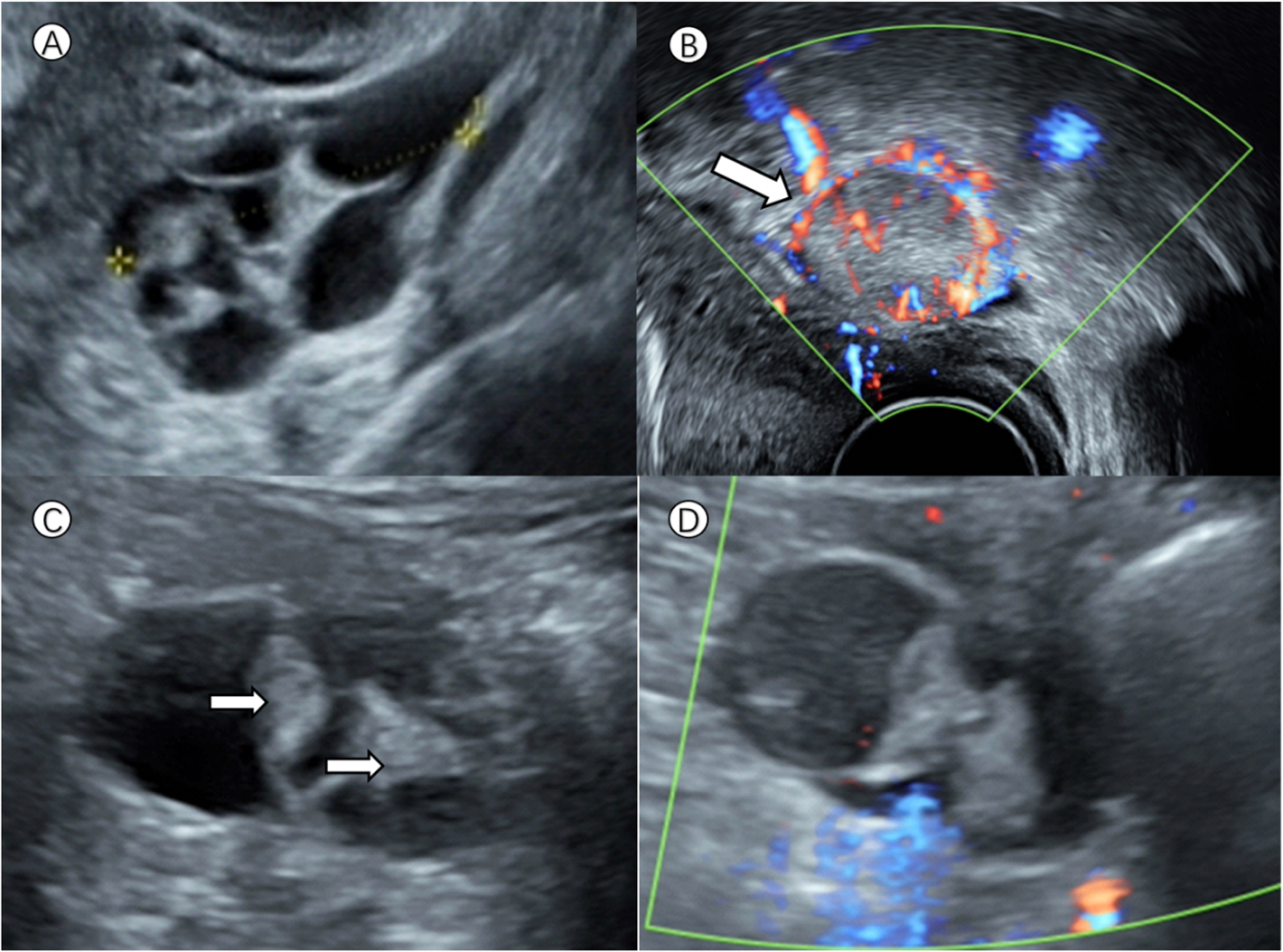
Greyscale and Doppler ultrasound images of the pelvic masses in a 39-year-old pregnant woman. a shows the period of the first trimester, and the cyst content was anechoic with thick septa. b, c and d were from the third trimester. b shows a solid hypoechoic area with rich blood flow signals in the Douglas pouch (white arrow). There were two papillary projections approximately 22 mm (arrows: c) with smooth contours and no vascularization on power Doppler (d)
